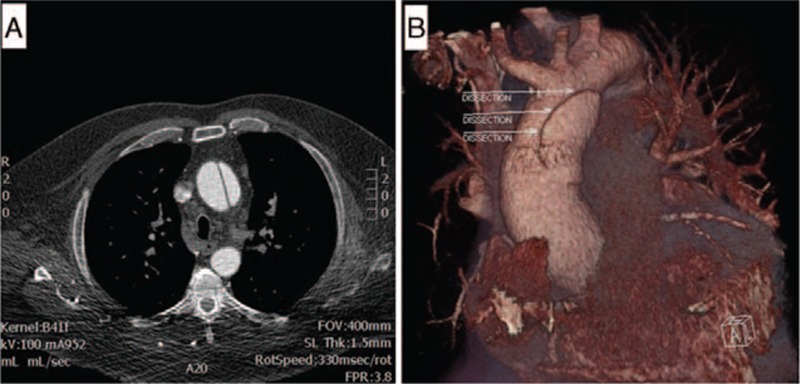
(A) Cross-sectional CT angiography showing a dissection flap in the ascending aorta. (B) 3D reconstruction displaying the same ascending aortic dissection.
